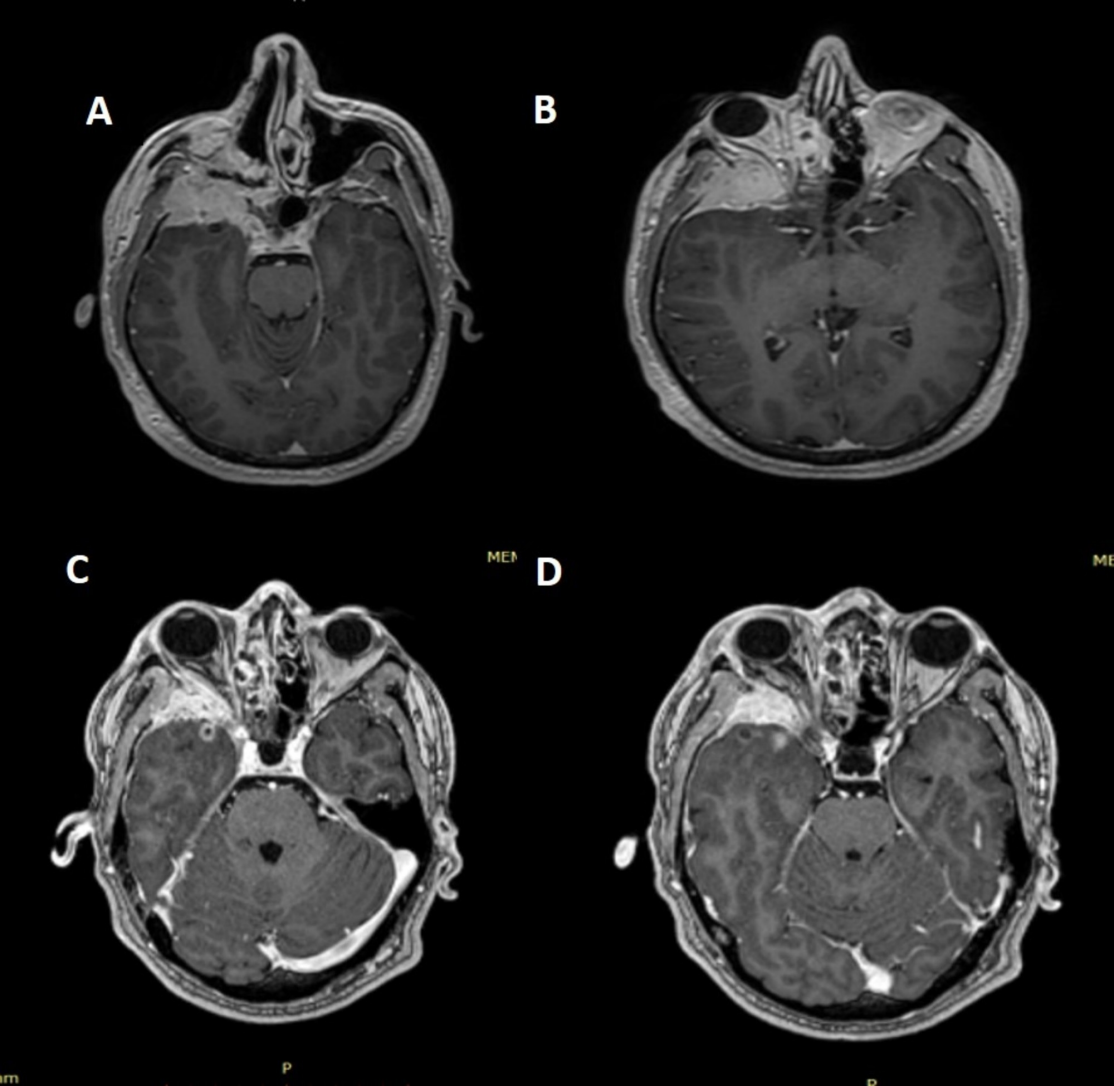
Magnetic resonance images (MRIs) before and after hypofractionated stereotactic radiotherapy 3 A-B: MRI at recurrence 3 C-D: MRI four months after radiotherapy
